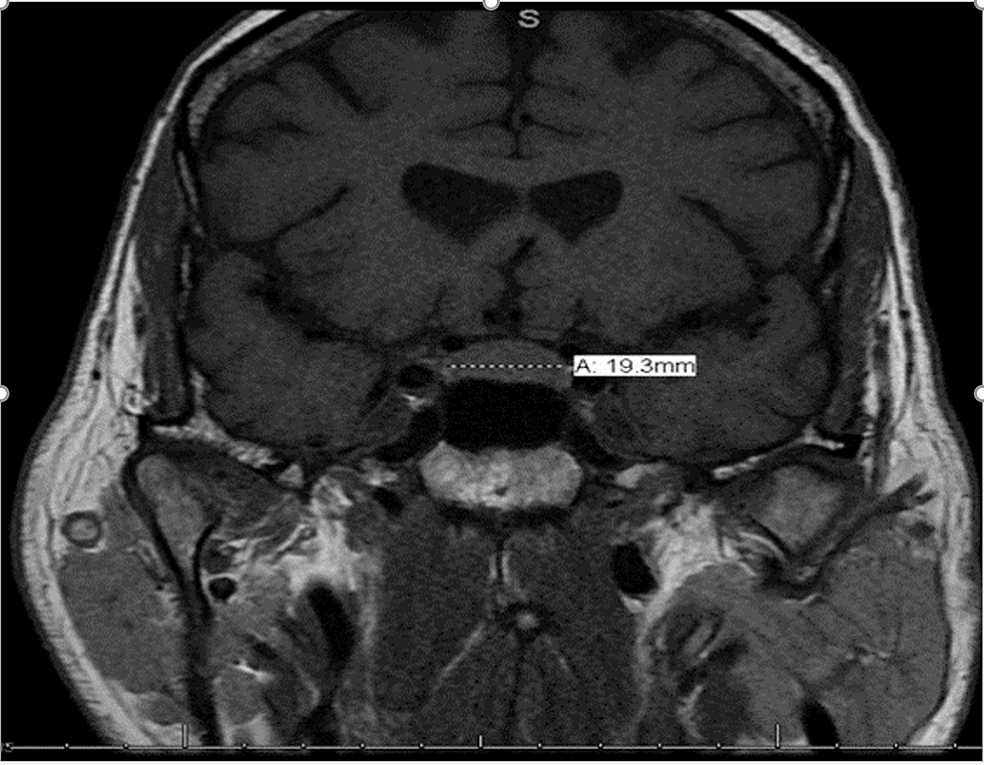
MRI brain showing pituitary gland enlargement in coronal section MRI: magnetic resonance imaging
